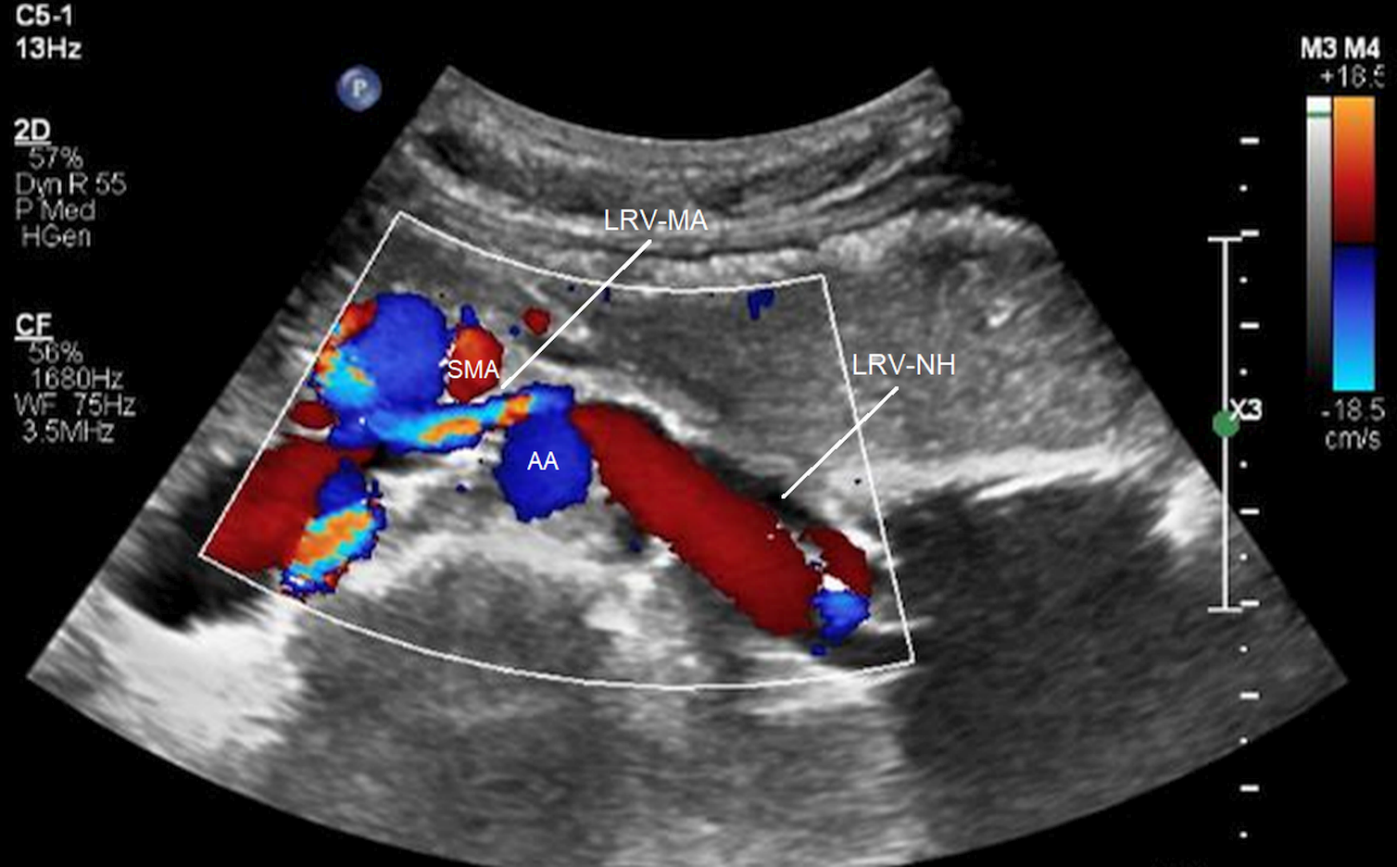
Compression of the left renal vein on color Doppler imaging. SMA, superior mesenteric artery; AA, abdominal aorta; LRV-MA, left renal vein between the SMA and AA; LRV-NH, left renal vein near the hilar [from: Kim (32). Reprinted with permission from Dr Kim].
